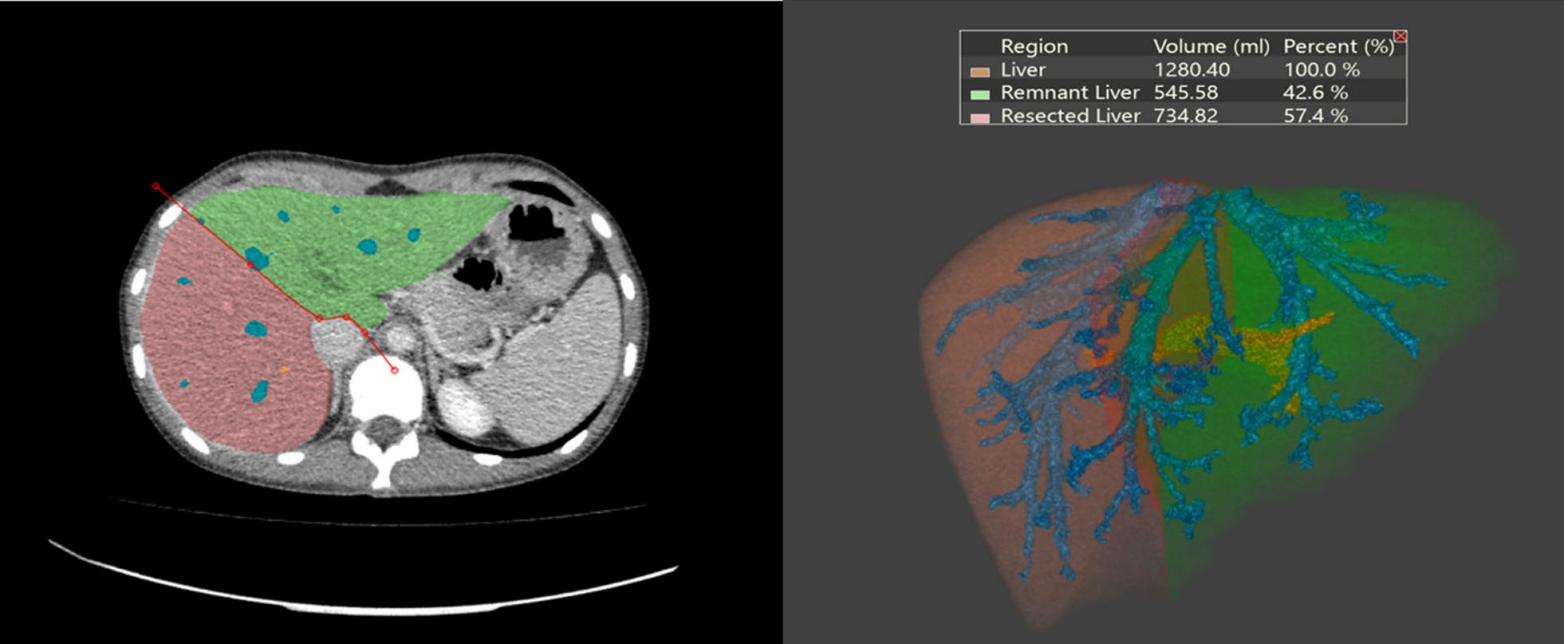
Automated CT volumetry and 3D resection plan by Vitrea software
